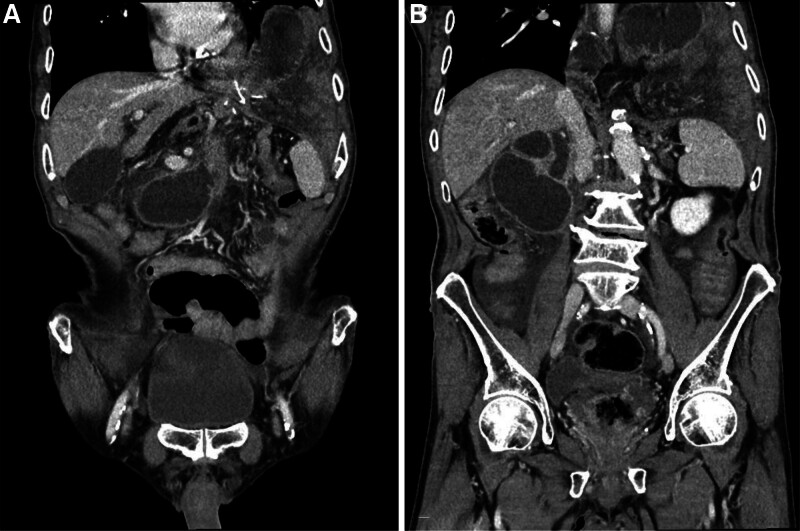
Abdomen–pelvis computed tomography before surgery revealing: (A) Distension of the afferent loop and hiatal hernia. (B) Distension of the gallbladder and common bile duct.
